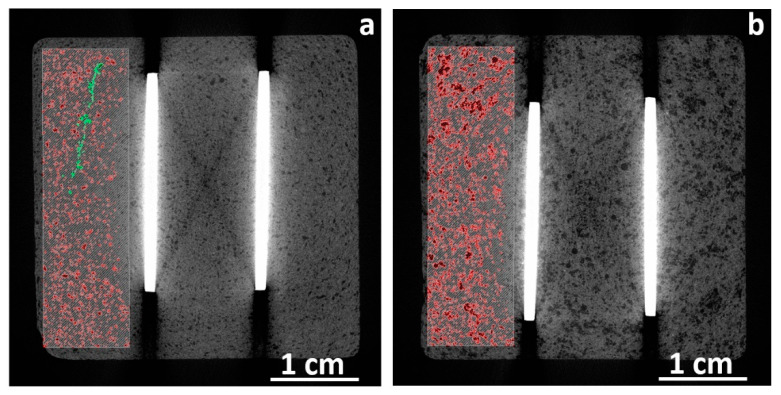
X-ray tomography cross-sections through samples K1 (a) and K2 (b). The red spots at the left side of the images indicate carbon nanofiber aggregates. The green pattern on figure (a) represents a fracture present in the material.
